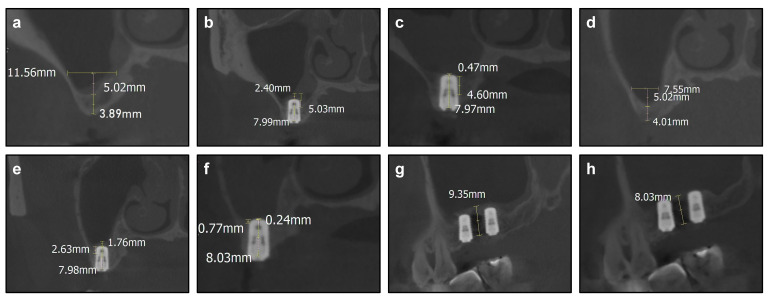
Cone-Beam Computed Tomography (CBCT) and measured data. (a) Residual bone height (RBH) of right maxillary first molar before elevation. (b) Height of the graft apically (aGH) of right maxillary first molar after elevation. (c) aGH of right maxillary first molar 6 months after elevation. (d) RBH of right maxillary second molar before elevation. (e) aGH of right maxillary second molar after elevation. (f) aGH of right maxillary second molar 6 months after elevation. (g) aGH between 2 implants after elevation. (h) aGH between 2 implants 6 months after elevation.
